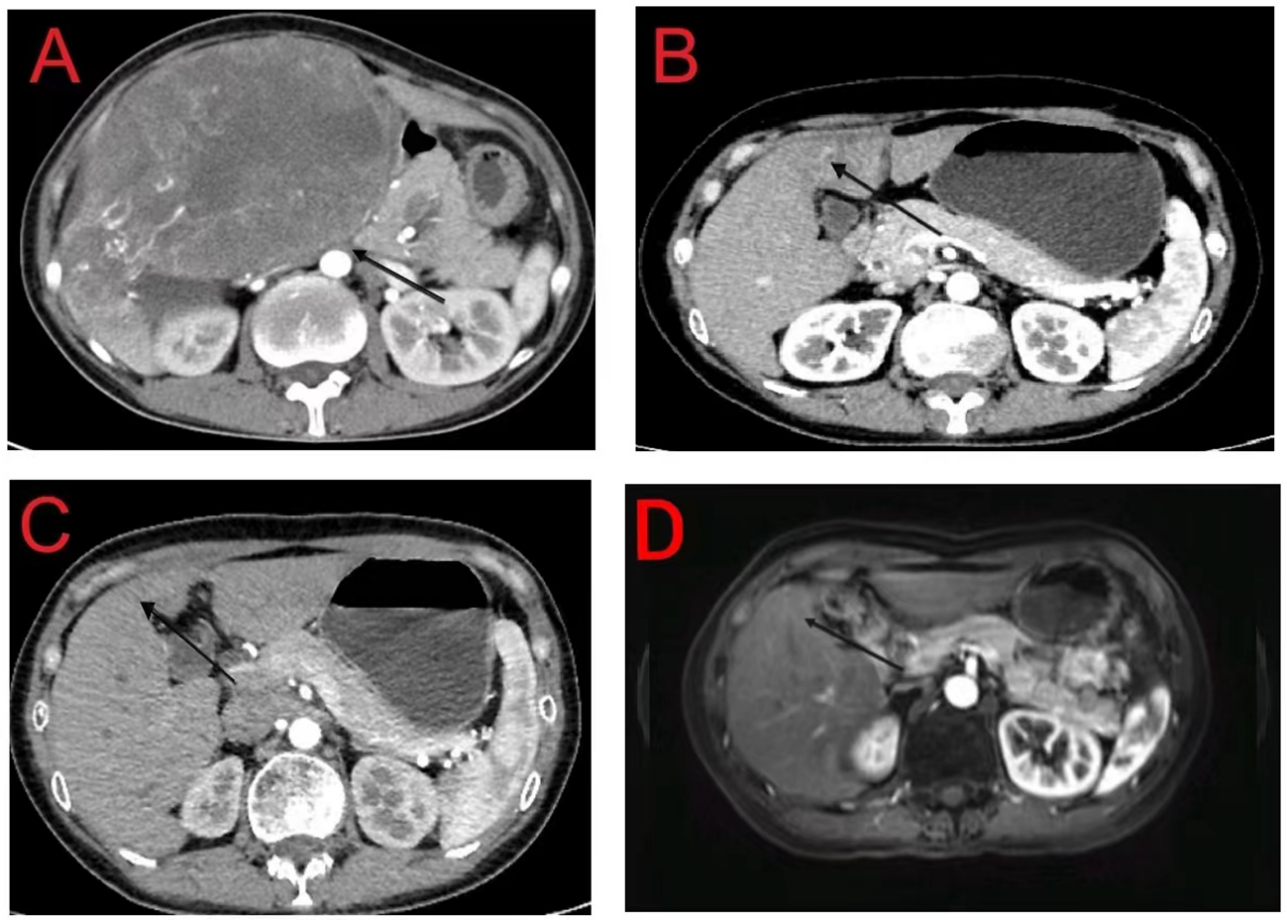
(A) Initial computed tomography (CT) scan of the abdomen showing a 160 mm × 110 mm hepatic mass with no daughter nodules in the other hepatic lobe or tumoral thrombosis in the inferior vena cava and right atrium; (B) Tumor mass is reduced to 37 mm × 31 mm following neoadjuvant treatment; (C, D) CT and magnetic resonance imaging reveal post-treatment changes in the junctional area of the left and right lobes of the liver with no recurrent lesions.
